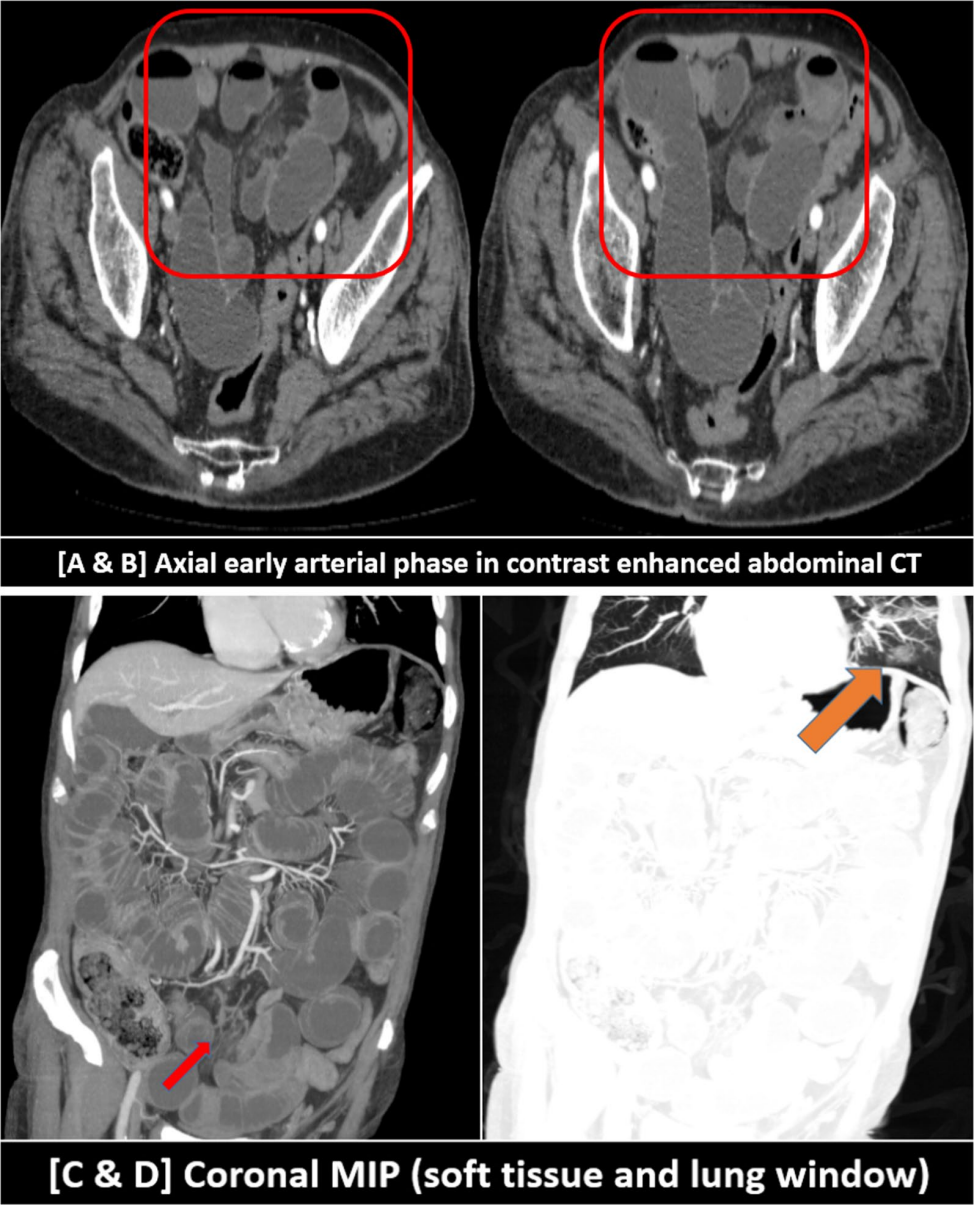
A 55-years-old male patient was proved for COVID-19 infection. He presented to the emergency unit during the second week of infection with severe abdominal pain and vomiting. Mesenteric CT arteriography and venography were performed without oral preparation. [A, B] Axial images of abdominal CT at the arterial phase revealed dilatation of the small bowel loops (ileus), meanwhile, some of the distal peripheral arcades of the superior mesenteric artery (SMA) are non opacified (red square) with stranding of the surrounding fat planes and minimal mural thickening of the supplied ileal loops. [C] Coronal image of abdominal CT at the arterial phase with MIP reconstruction demonstrated the severely attenuated distal SMA arcades inferiorly. [D] Coronal image (lung window) at the same left showed left basal ground-glass COVID-19 pneumonic patch
